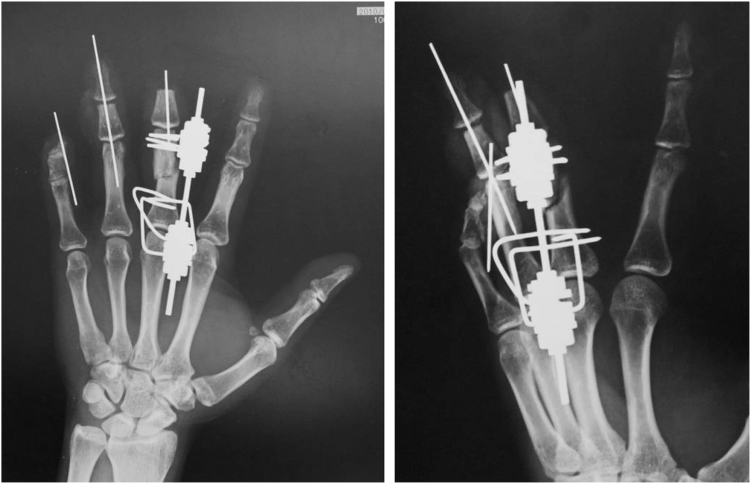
X-ray after application of Ilizarov minifixator.
